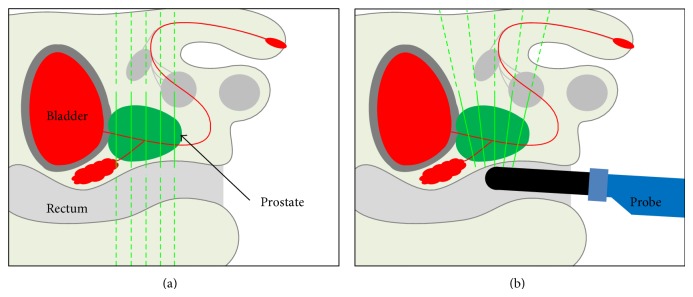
Different planes (yellow lines) complicate visual registration. (a) MRI and (b) TRUS slice the 3D prostate volume into different planes of 2D images, based upon different and variable orientations.
